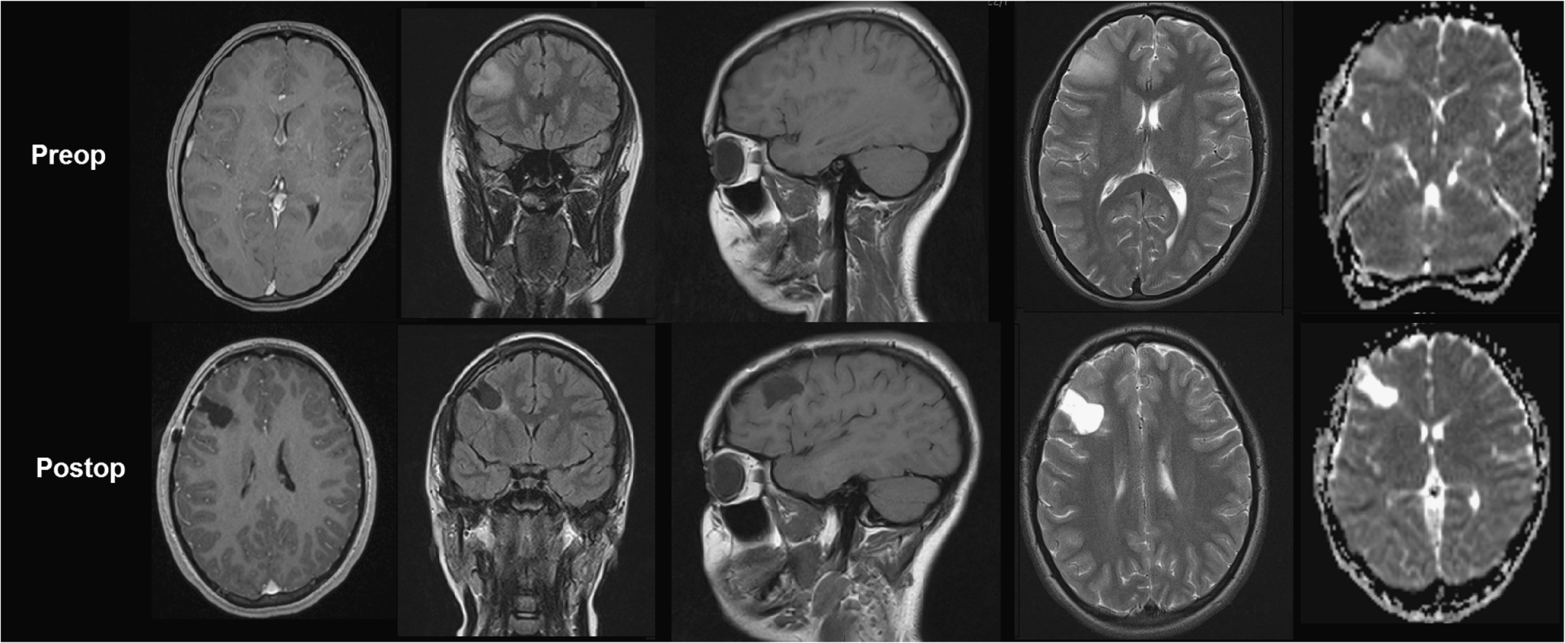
Preoperative and 3-month postoperative MRI images from VF including (*from left to right*) axial T1 post-gadolinium, coronal FLAIR MRI, sagittal T1, axial T2 and axial apparent diffusion coefficient (ADC) maps
